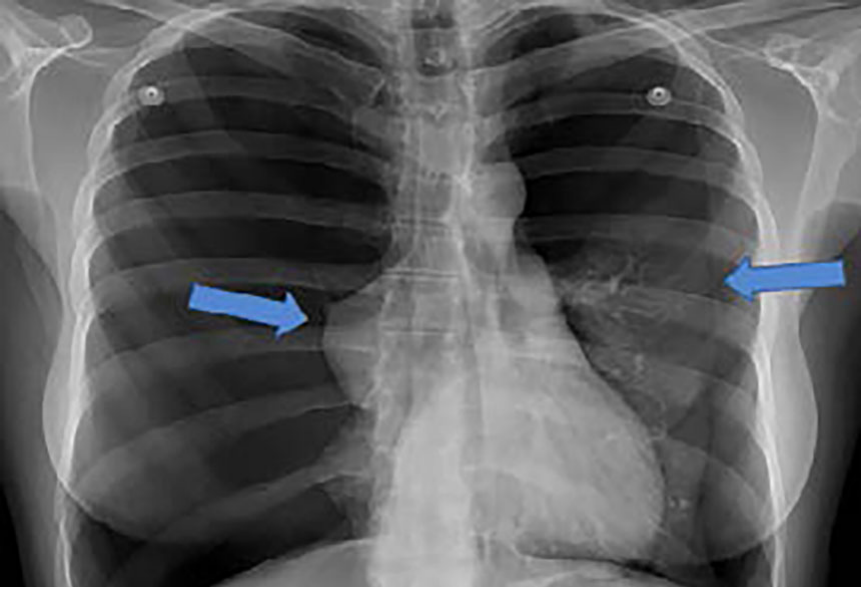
Posterior-anterior chest radiograph with bilateral pneumothoraces. Lung marking indicated by arrows.
